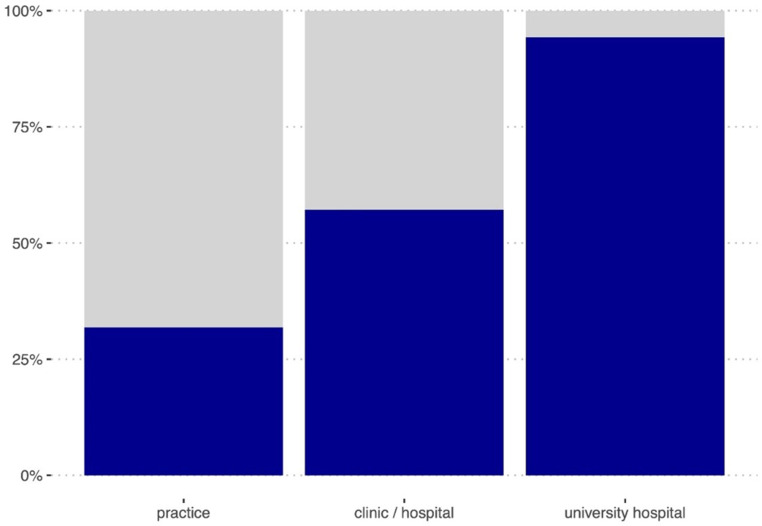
Access to PET/CT stratified by working place. Q22: “I have access to PET/CT within my department/practice network/clinic/university hospital.” Participants answering “yes”: *blue* part of the column; “no”: no data, *grey*; see also Table 2
